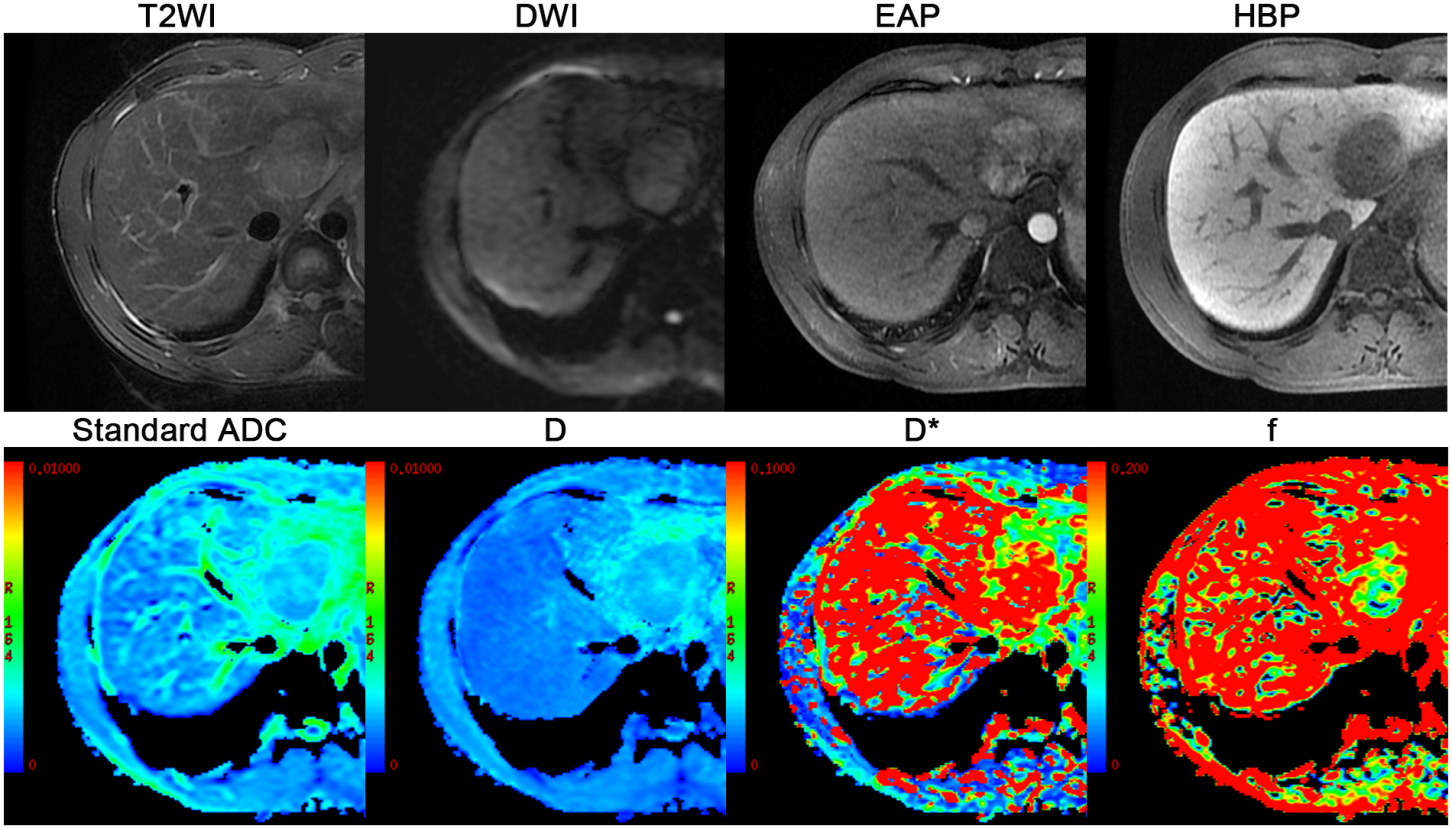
A 28-year-old man with chronic hepatitis B and who underwent liver resection for HCC (moderately differentiation) without microvascular invasion. MRI shows a 4.8 cm hyperintensity on axial respiratory-triggered, T2-weighted, fast-spin-echo (FSE) image (T2WI) in hepatic II with hyperintensity on DW imaging and early arterial-phase(EAP) and a definite hypointensity on hepatobiliary-phase(HBP) images, presented with a regular shape. Corresponding IVIM-derived four parameters (Standard ADC, D, D* *f*) maps, the value of Standard ADC, D, D* f for the lesion was 2.10 × 10^−3^ mm^2^/sec, 1.93× 10^−3^ mm^2^/sec, 83.5× 10^−3^ mm^2^/sec,14.7%, respectively.
